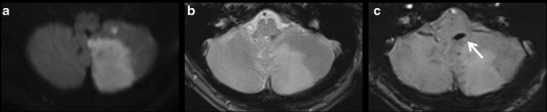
A 48-year-old patient presenting sudden-onset vertigo. DWI (a), T2 spin-echo (b), and SWI (c) sequences in the axial plane. a Acute ischaemic lesion in the territory of the left posterior inferior cerebellar artery. b No intra-vascular signal anomaly. c Susceptibility vessel sign revealing an intra-arterial thrombus in the left posterior inferior cerebellar artery
